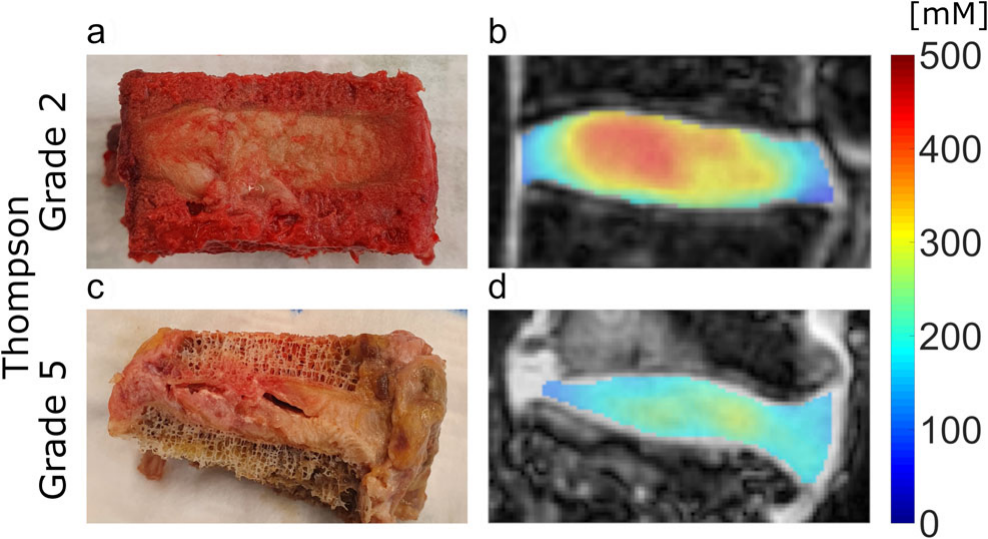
(a, c) Anatomical images for Thompson grading and (b, d) aTSC overlays onto the acquired ^1^H MRI images. Figure (a) shows an intervertebral disc (IVD) with a Thompson grade of 2 and a mean aTSC of 371.7 ± 42.4 mM for the NP, 258.7 ± 37.4 mM for the AF, and 298.0 ± 92.7 mM for the whole IVD. Figure (c) shows an IVD with a Thompson grade of 5 and a mean aTSC of 201.1 ± 45.8 mM for the NP, 163.0 ± 46.6 mM for the AF, and 169.2 ± 48.5 mM for the whole IVD. Figures (b) and (d) present the corresponding MR images.
